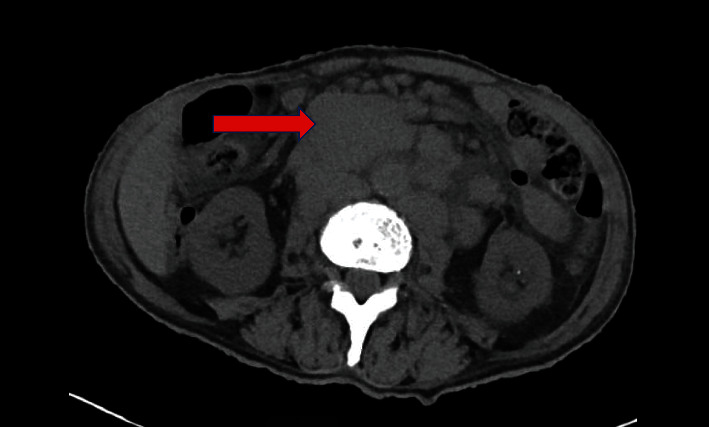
CT of the abdomen, axial view, showing a soft tissue mass anterior to the abdominal aorta and posterior to the body of the pancreas measuring 8 × 6 × 4 cm (red arrow) representing lymphadenopathy from the diffuse B cell lymphoma.
